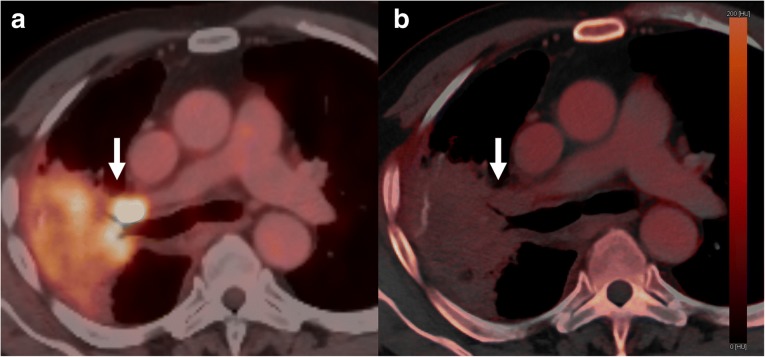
PET and DECT images comparison in a patient with lung cancer and metastatic right hilar adenopathy. a Fused PET/CT dataset showing metabolic uptake of the right hilar adenopathy and lung mass (*arrow*). b Dual-energy iodine map dataset showing iodine uptake in the same region (*arrow*)
